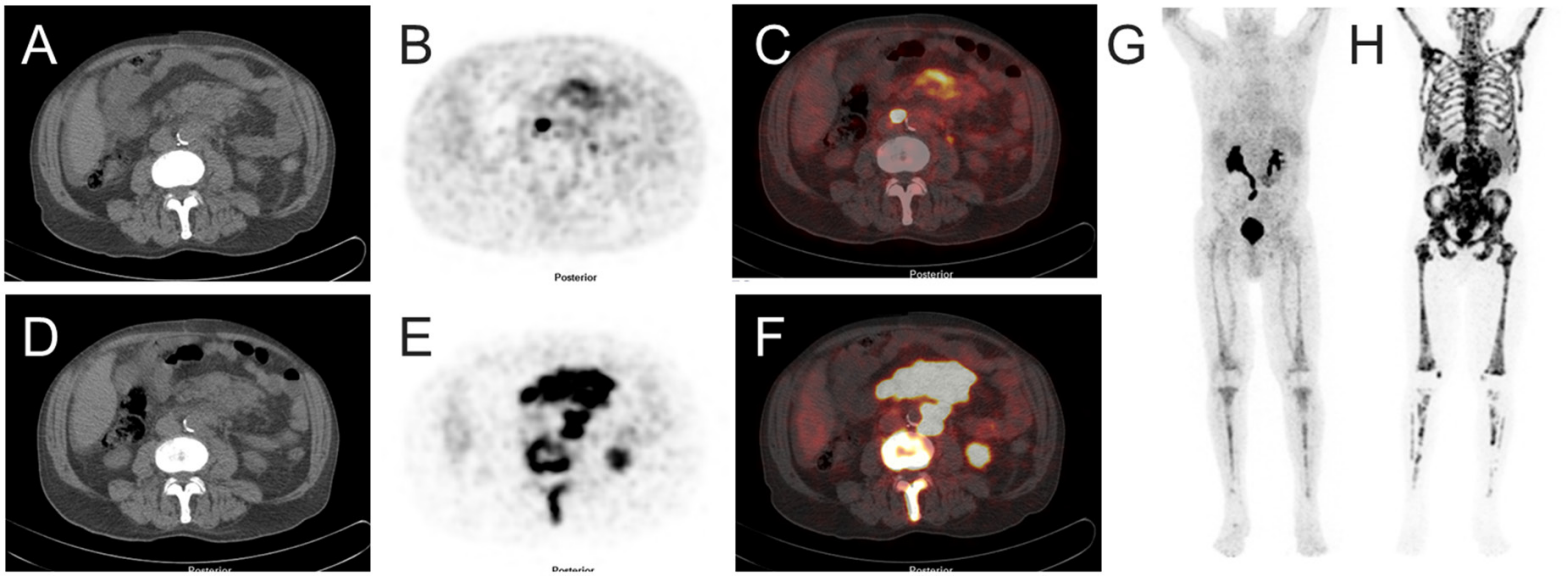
Image example of a clinically indicated [^68^Ga]Pentixafor PET/CT scan of a 74 year old patient with castration-resistant metastasized prostate cancer after all approved systemic therapies [^68^Ga]Pentixafor shows only faint or no increased uptake in disseminated nodal and bone metastases (whereas metastases are highly PSMA-positive on [^68^Ga]PSMA PET/CT) Transaxial sliced of (A) CT, (B) [^68^Ga]Pentixafor PET, (C) fused [^68^Ga]Pentixafor PET/CT and (D) CT, (E) [^68^Ga]PSMA PET, (F) fused [^68^Ga]PSMA PET/CT; maximum intensity projection of (G) [^68^Ga]Pentixafor PET and (H) [^68^Ga]PSMA PET.
